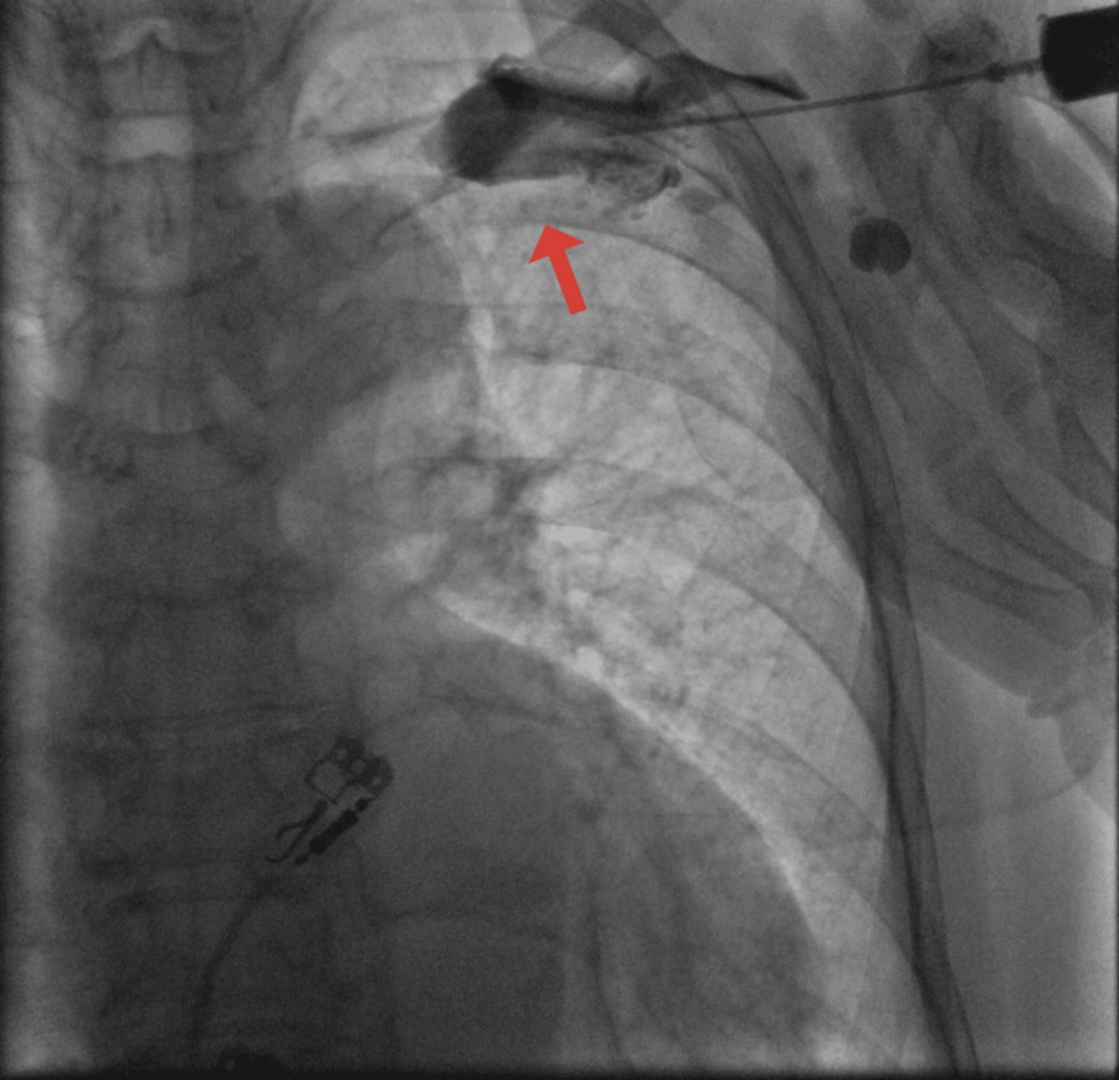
Chest X-ray angiography with contrast shows a thrombosed subclavian vein on the left side.
